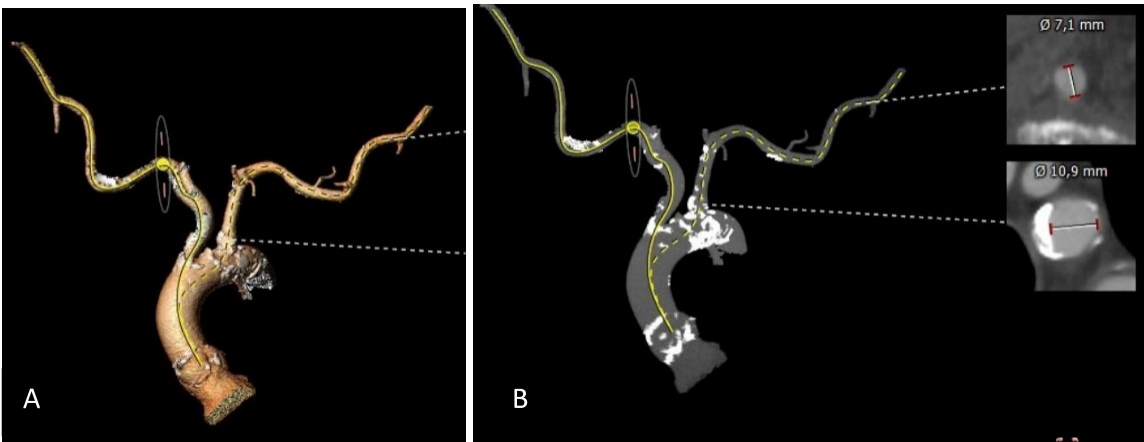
Computed tomography images in preparation for a transaxillary
